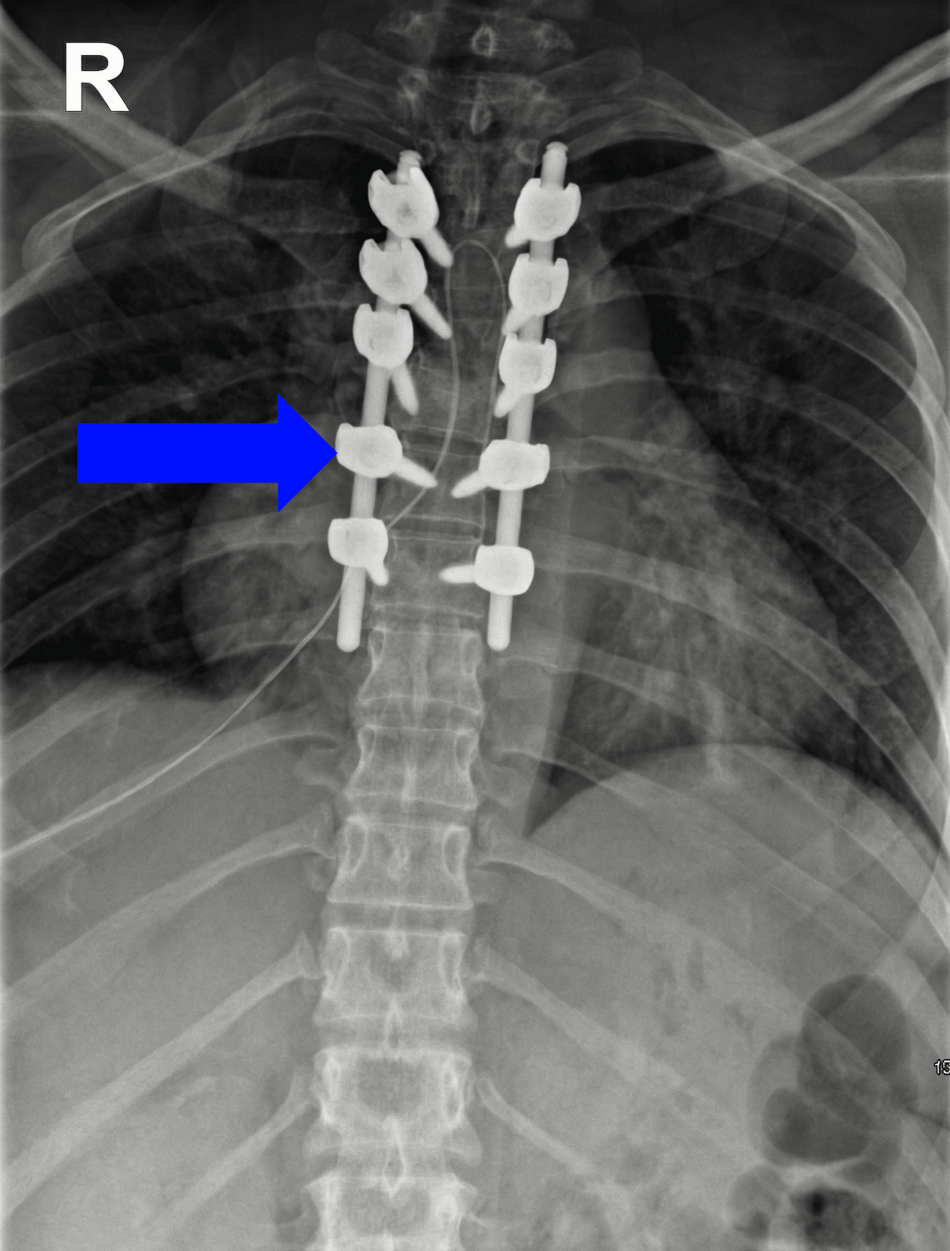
Postoperative anteroposterior (AP) radiograph of the thoracic spine
